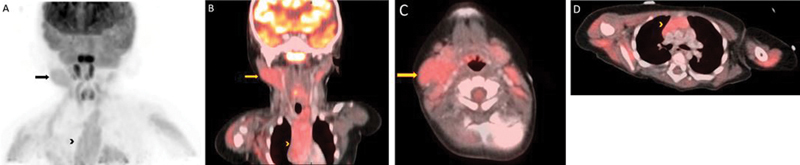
The PET/CT demonstrates a mildly FDG-avid submandibular mass (
